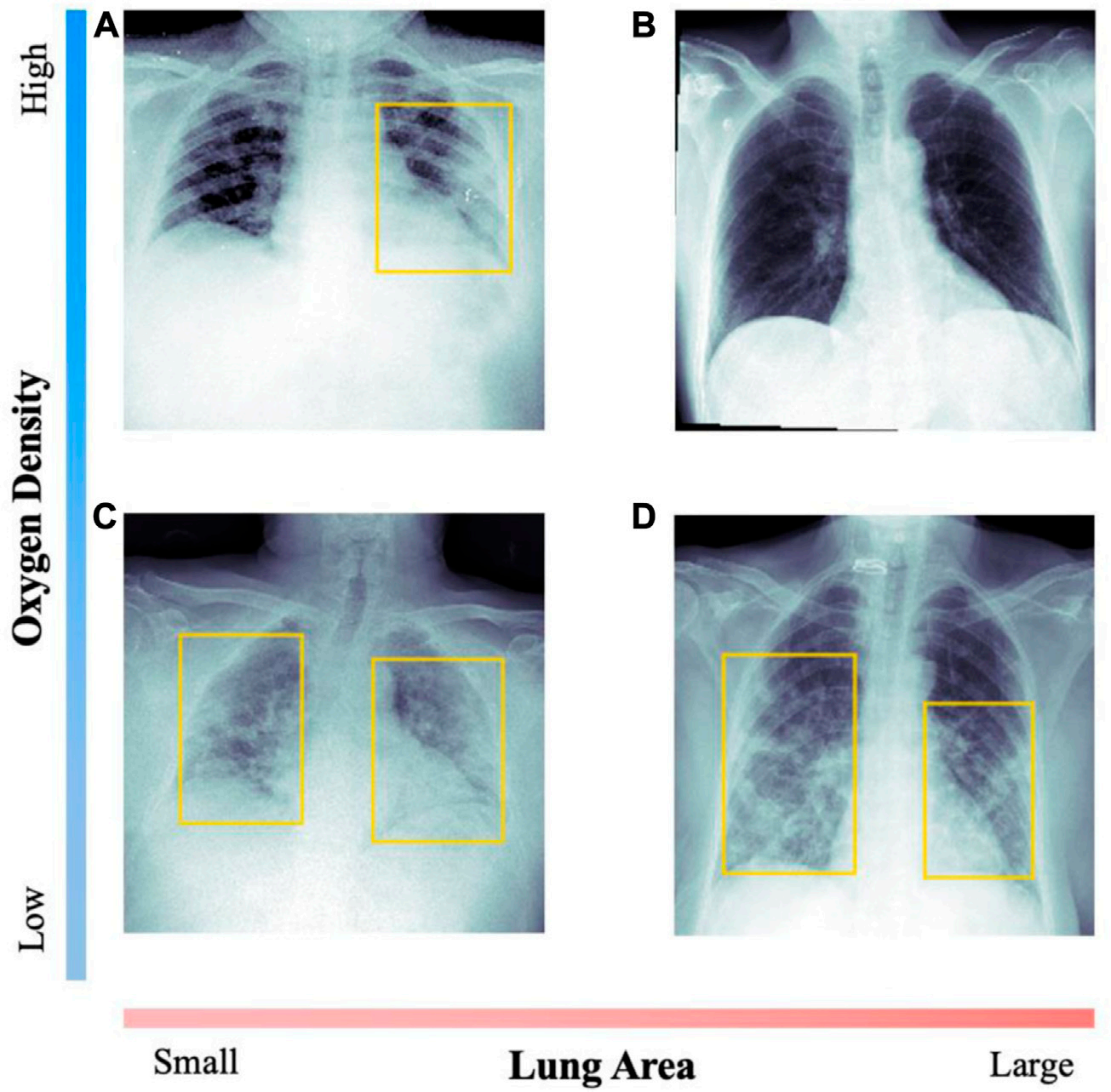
Comparison of radiographs with opposed values on the diagnosis variables. For each image, the patient’s data is included. Yellow boxes indicate lung opacity detection. Image (A) corresponds to a 47-year-old woman, with medium saturation; (B) to a 53-year-old man with adequate saturation; (C) to an 82-year-old man with extreme saturation; (D) to a 72-year-old woman with low saturation.
